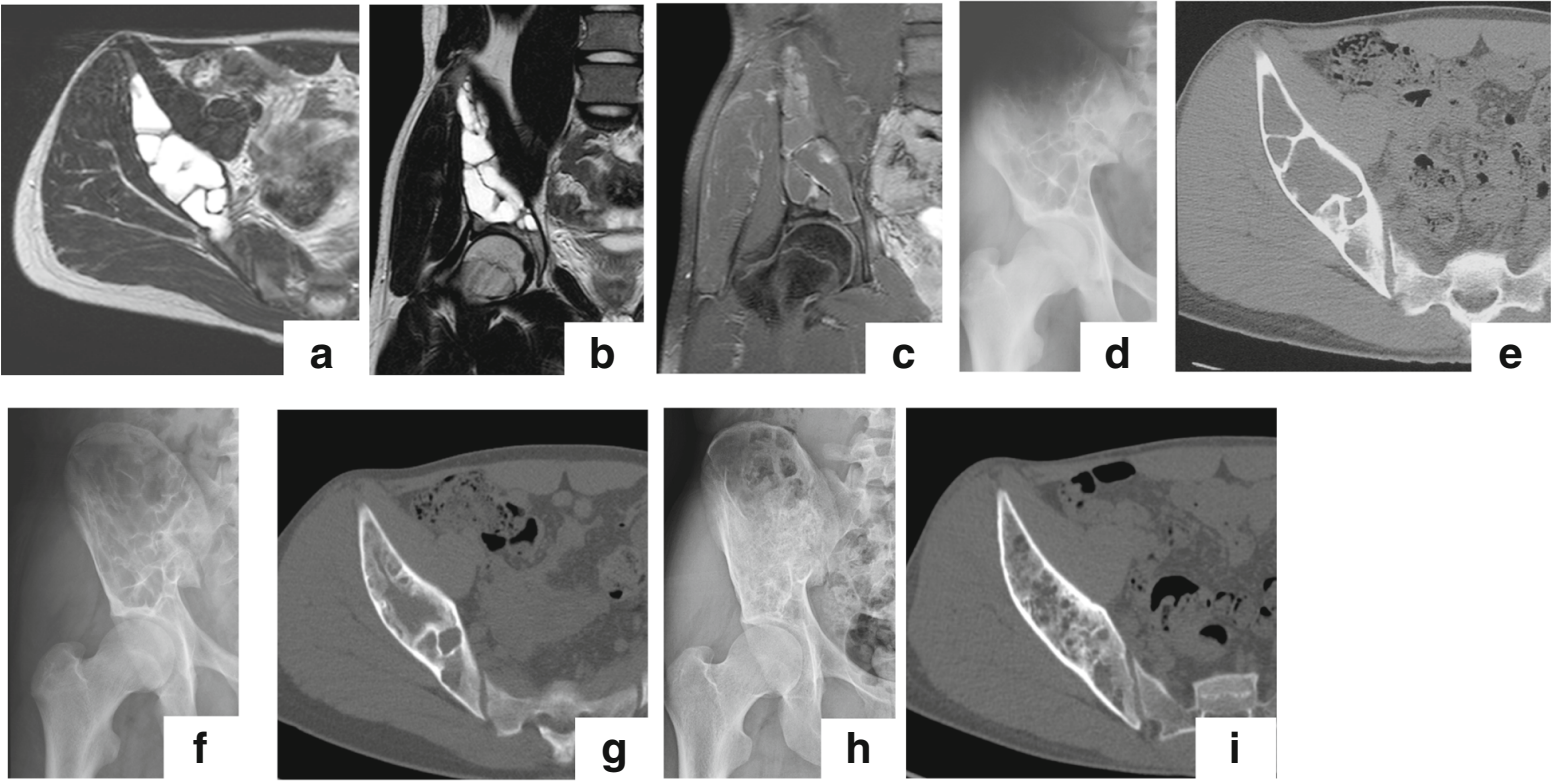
Typical case of a suspected ABC treated with ESC. Magnetic resonance (MR) images of a 16-year-old boy who presented with bruising of his hip sustained during contact sports participation: (a) axial T2; (b) coronal T2-weighted; and (c) coronal T1-weighted enhanced with gadolinium (Gd). The plain radiograph is shown in (d). The preoperative computed tomography (CT) image (e) shows the bone lesion with cortical thinning extending from the iliac wing to the periacetabular area. Plain radiographs after surgery (f). CT image at 6 months after ESC (g) shows remodeling of the cystic lesion by cancellous bone. At 6 years after ESC (h), the pelvic bone was fully remodeled despite a residual cyst and without any symptoms (class B) (i)
